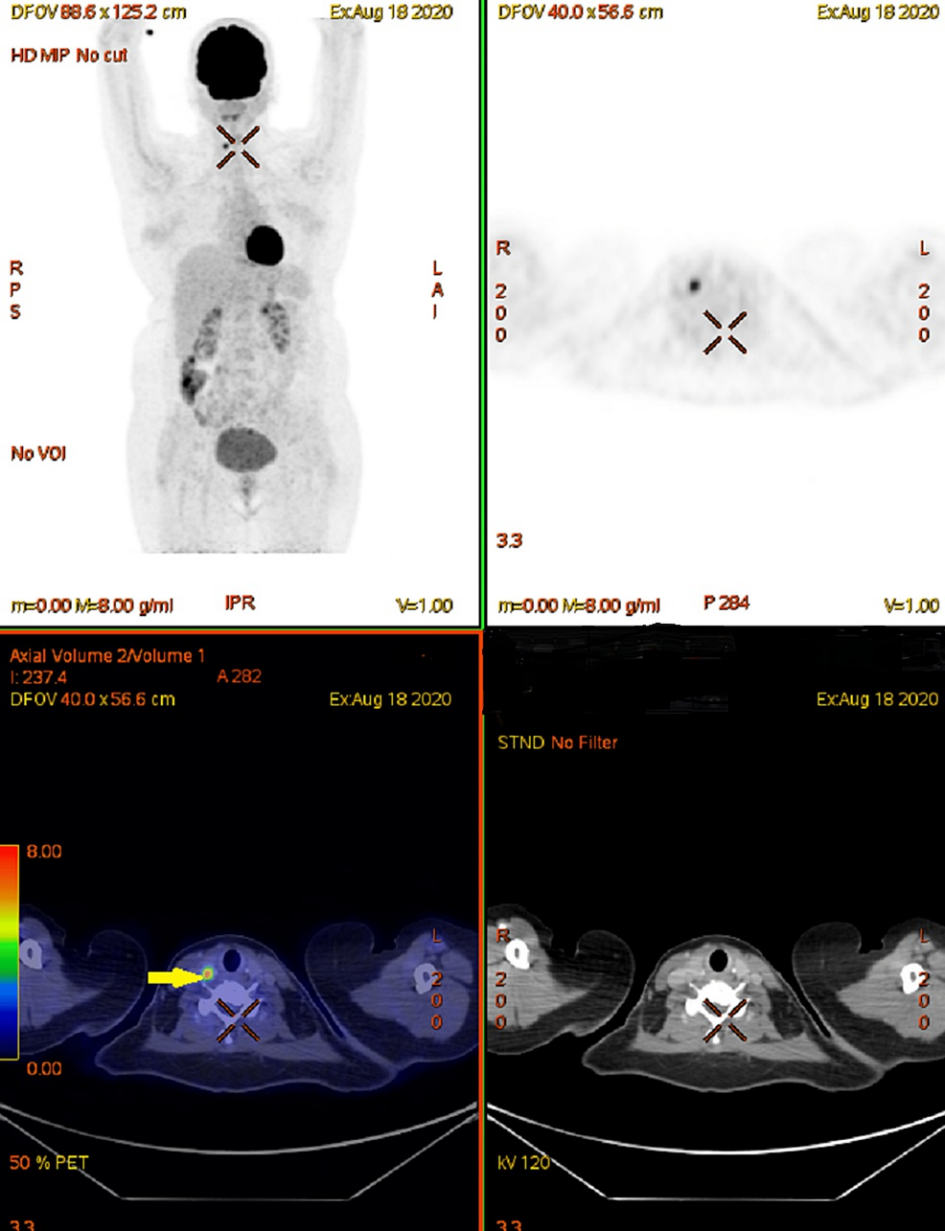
Post-chemotherapy PET-CT scan images The images are showing a complete metabolic response of lymph nodes with right thyroid uptake (yellow arrow).
